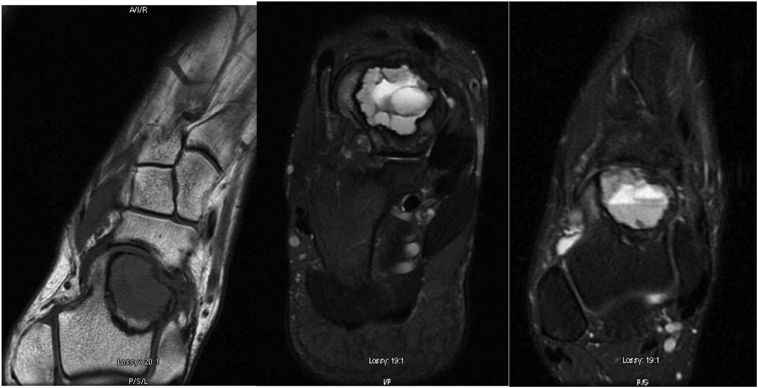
(A, B, C): Coronal T1, Axial T2, Coronal SE MRI images. Post-recurrence MRI showed a multiloculated expansile cystic lesion within the neck and head of the talus measuring 2.6 × 2.3 × 2.1 cm with a well-defined sclerotic margin correlating with the known chondroblastoma-ABC. Minor adjacent osseous oedema was present which had diminished significantly when it was compared with the prior pre-operative MRI. Otherwise, there were no suspicious features or significant new findings apparent.
